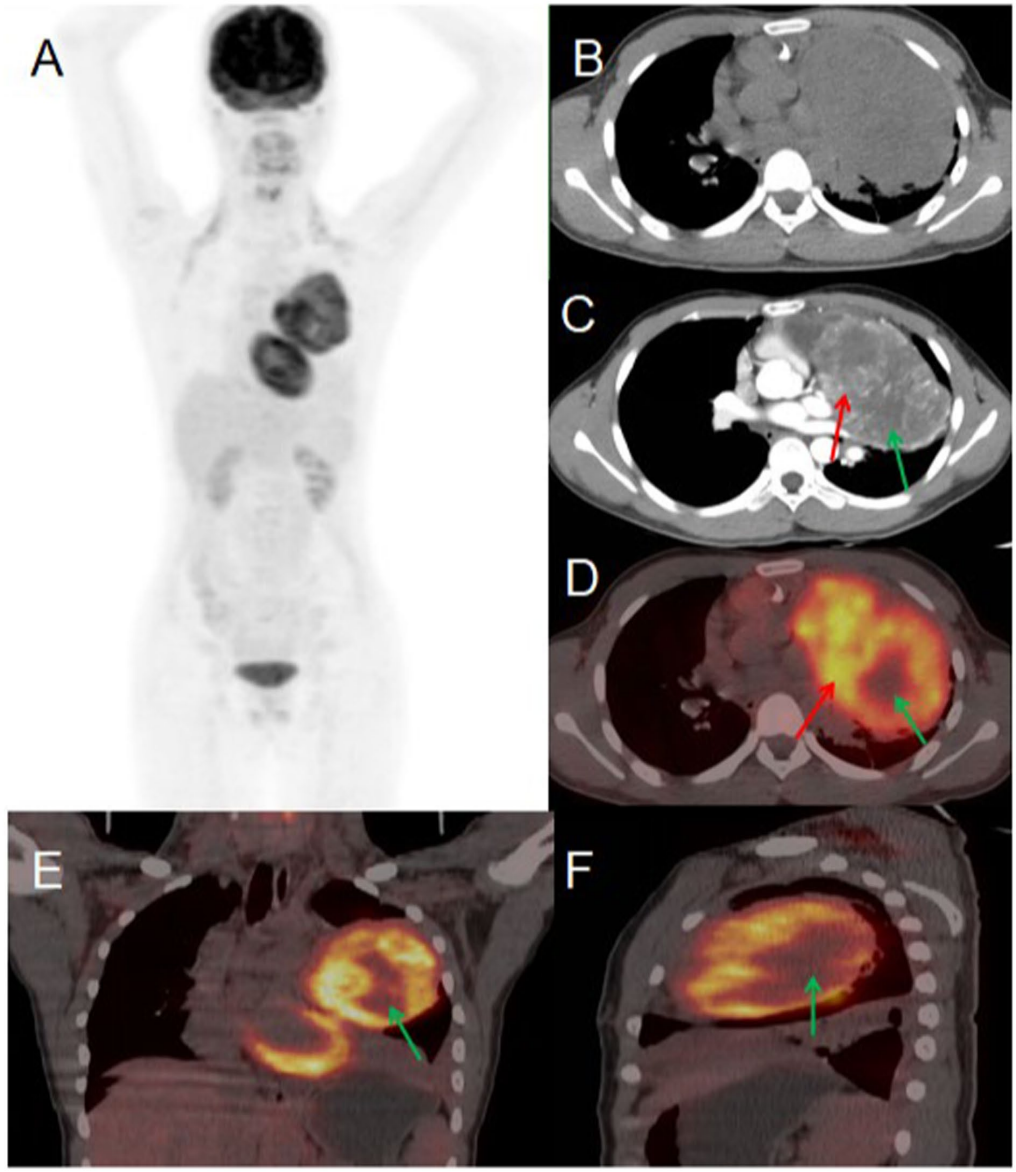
Female, 56 years old, malignant primary pulmonary solitary fibrous tumors. (A) Whole body MIP. (B) Axial, non-contrast-enhanced CT. (C) Axial, enhanced CT-arterial phase. (D) Axial fusion. (E) Coronal fusion. (F) Sagittal fusion. Contrast-enhanced CT findings: an approximately 81 × 101 mm soft tissue mass was identified in the left upper lobe, demonstrating: heterogeneous but significant enhancement in the solid components (red arrow, C). Non-enhancing areas corresponding to necrosis and cystic degeneration (green arrow, C). ^18^F-FDG PET/CT findings: the left upper lobe mass displayed: prominent necrotic-cystic degeneration throughout the lesion. Markedly increased FDG avidity in the solid components (SUV_max_ 9.6; red arrow, D). Absence of significant FDG uptake in the necrotic-cystic areas (green arrow, D–F).
